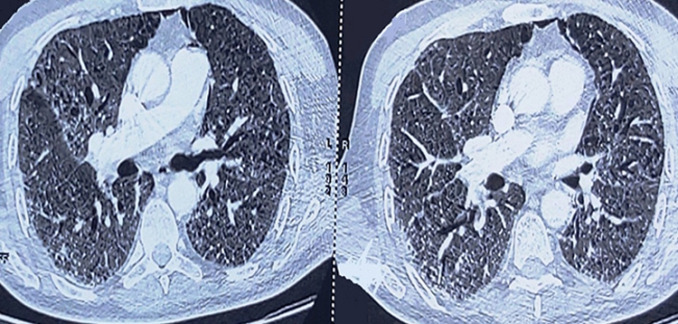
a chest CT-scan showing bilateral condensation and ground glass opacities in favor of an infectious pneumonia
